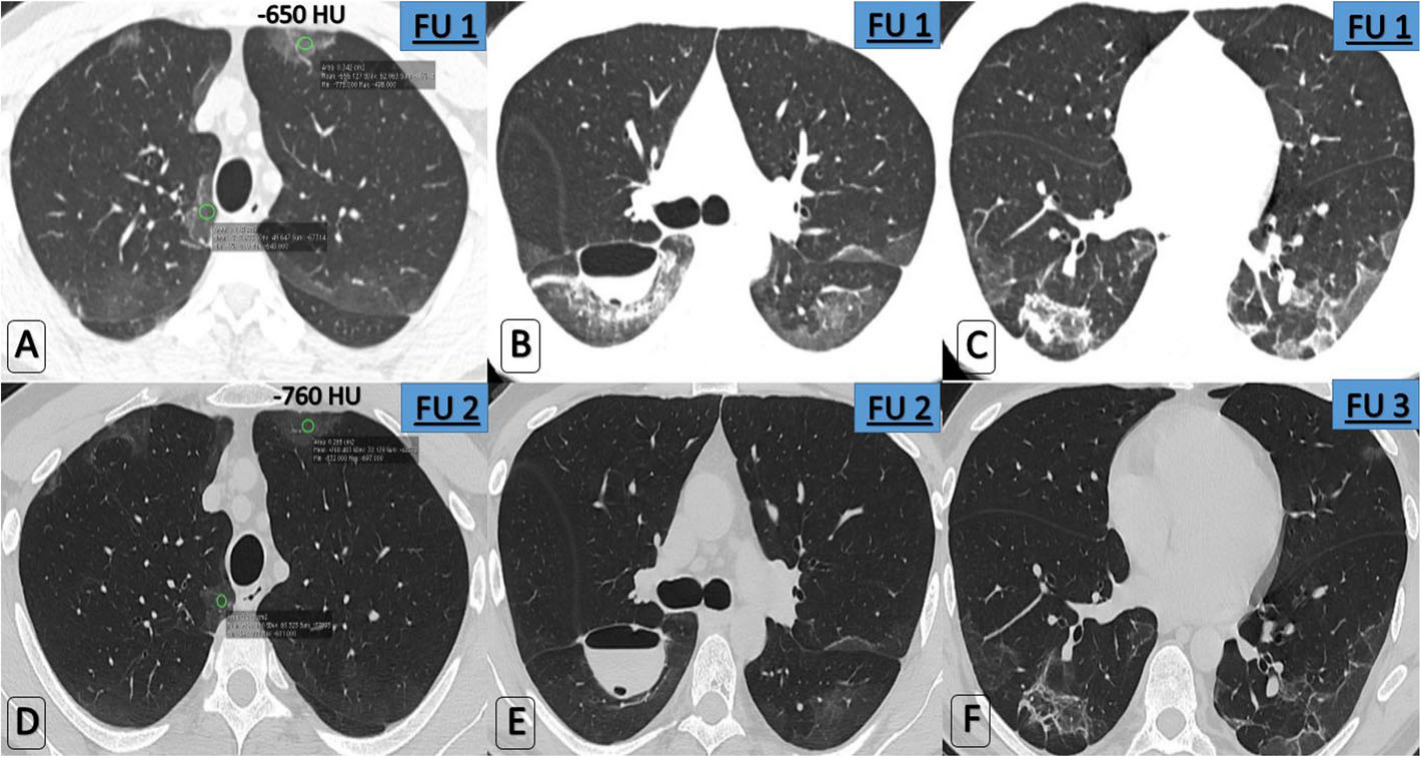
Serial follow-up for a completely asymptomatic recovered 44-year-male patient proved with COVID-19. a, b, c First follow-up study edited 4 days after two consecutive negative tests’ results announcing recovery. Axial HRCT chest—lung window; (a) higher GG attenuation (−650 HU), (b) process was complicated by encysted fissural hydro-pneumothorax surrounded by dense GGO and consolidative changes, (c) Peripheral dense organization is noted “Atoll sign.” d, e, f Next follow-up study edited 24 days later with axial HRCT chest—lung window; (d) GGO is approximating normal lung parenchyma (−760 HU), (e) Near-total resolution of the dense GGO and consolidations surrounding the encysted fissural collection which retained the same size, however, noticed increased fluid component and decreased internal air, (f) disappearance of the “Atoll sign” and lower GGO values. Laboratory tests were unremarkable and O_2_ saturation in room air was 96%. Clinical decision remains unchanged: No need for re-isolation or re-hospitalization
